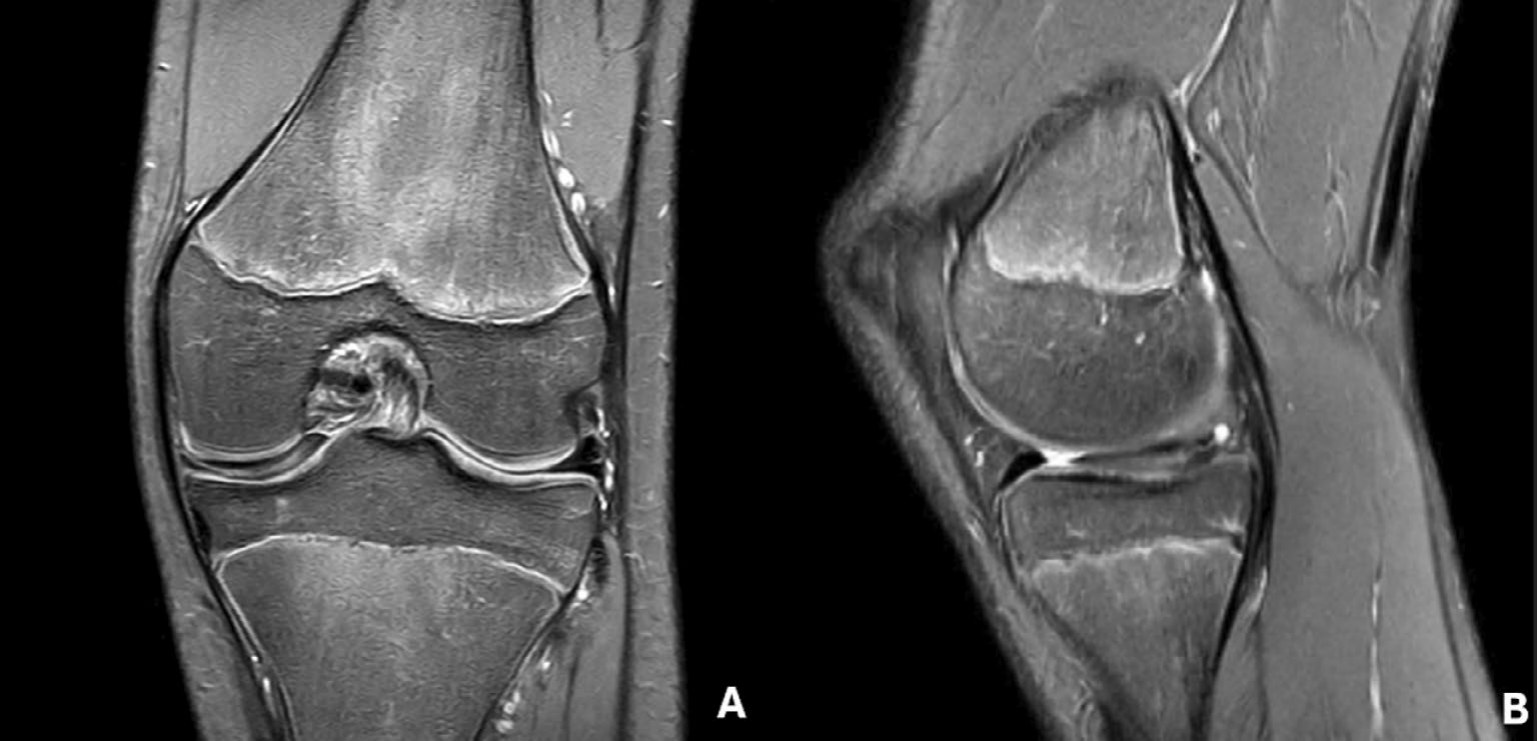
MRI of the left Knee. The coronal T2‐weighted scan shows a discoid medial meniscus, along with a degenerative horizontal cleavage tear (A) The tear extends peripherally dorsomedial in the area of the junction of the posterior horn and the corpus fossa, contacting the base with a parameniscal cyst of 3 mm as well as the surface with incipient displacement of both meniscal layers (B).
